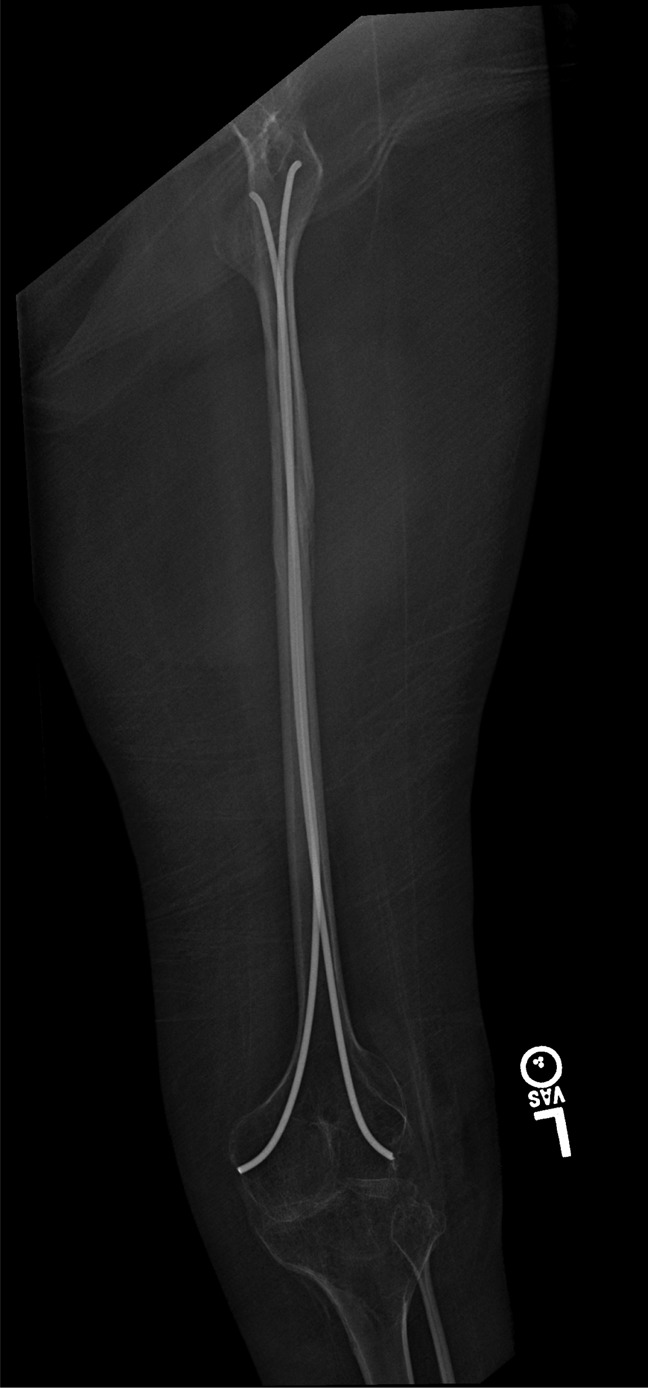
Six-month postoperative radiographs demonstrating full fracture healing with retained implant.
